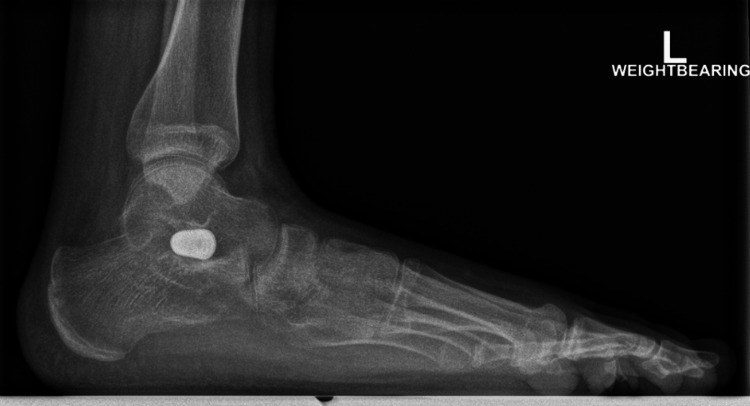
A post-operative weight-bearing lateral radiograph of the left foot, demonstrating improvements in the calcaneal pitch and Meary's angle
